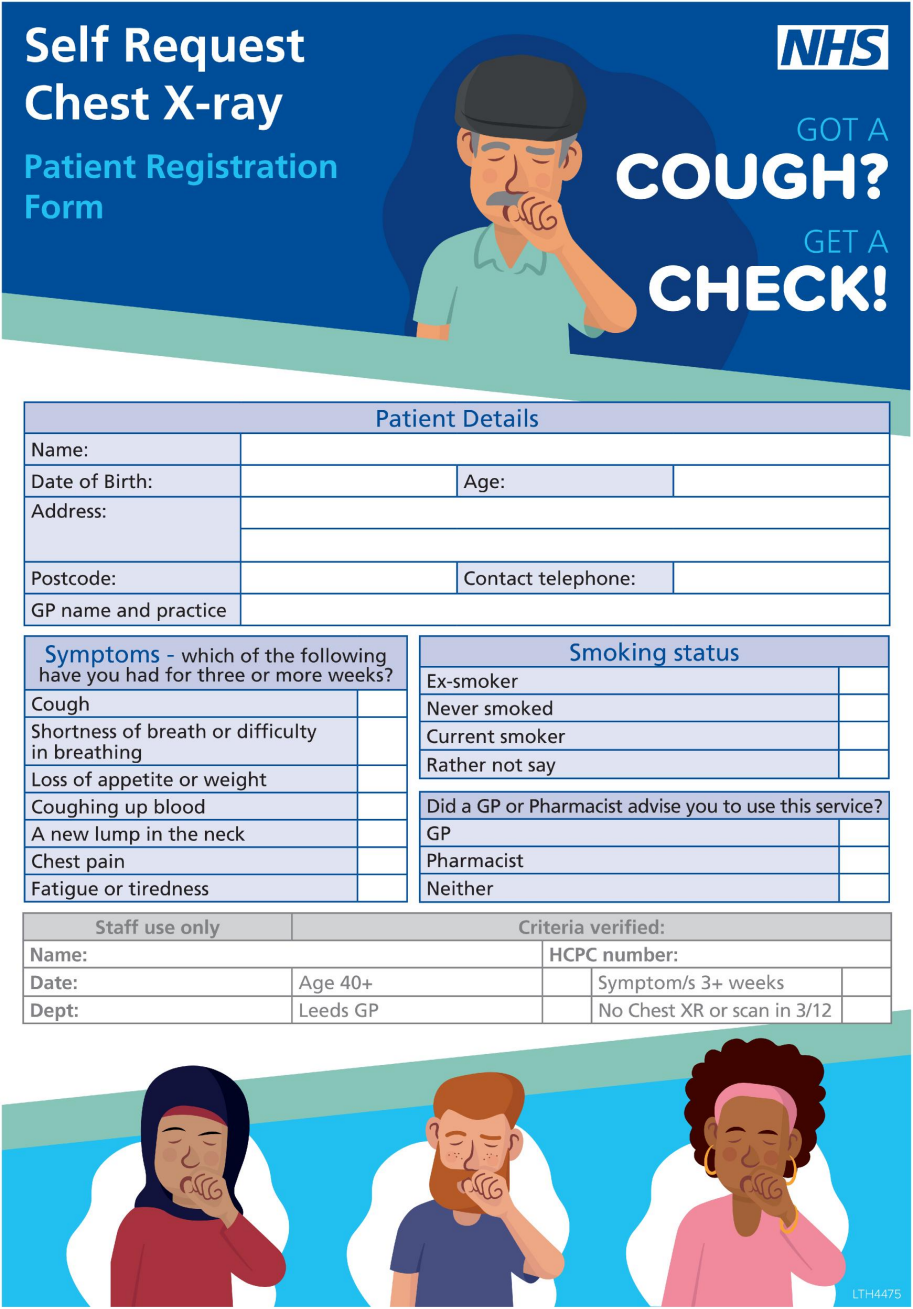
Patient registration form for the self-request chest x-ray service.
